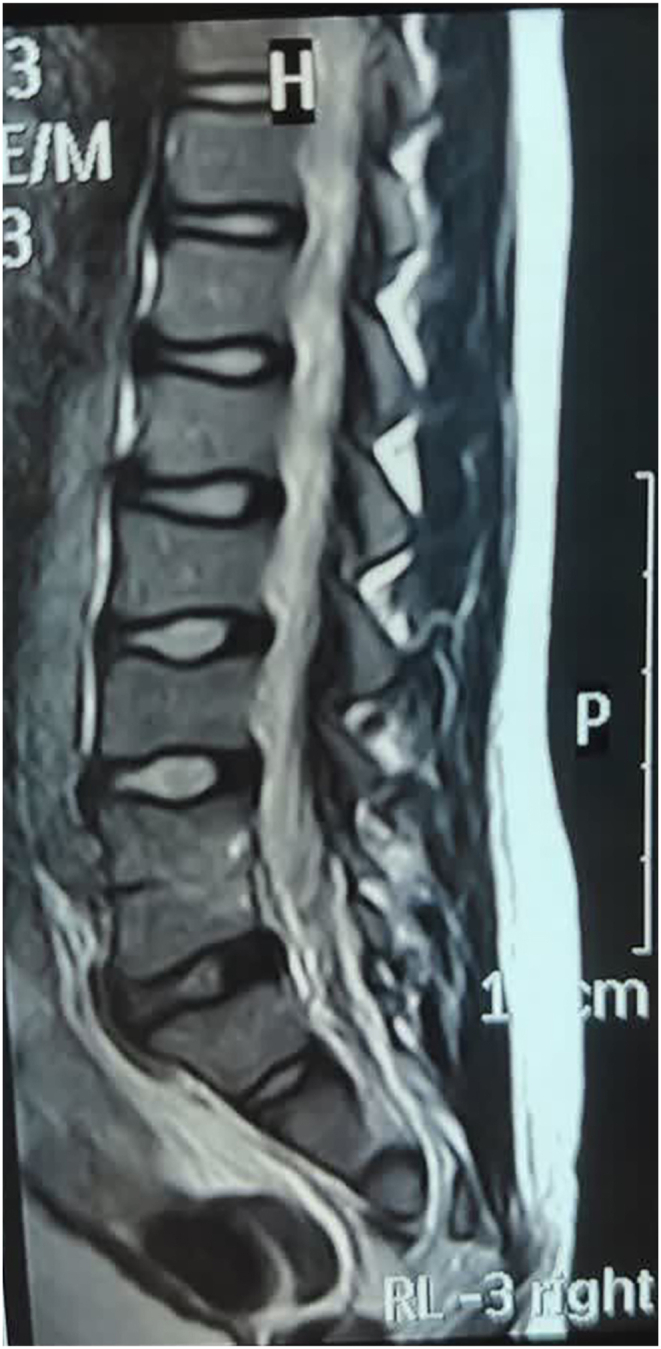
Sagittal and axial T2-weighted magnetic resonance imaging (MRI) demonstrates partial fusion of the L3 and L4 vertebral bodies (congenital block vertebra), with associated reduction in intervertebral disc height. The spinal cord terminates at a normal level without evidence of tethering or syringomyelia.
